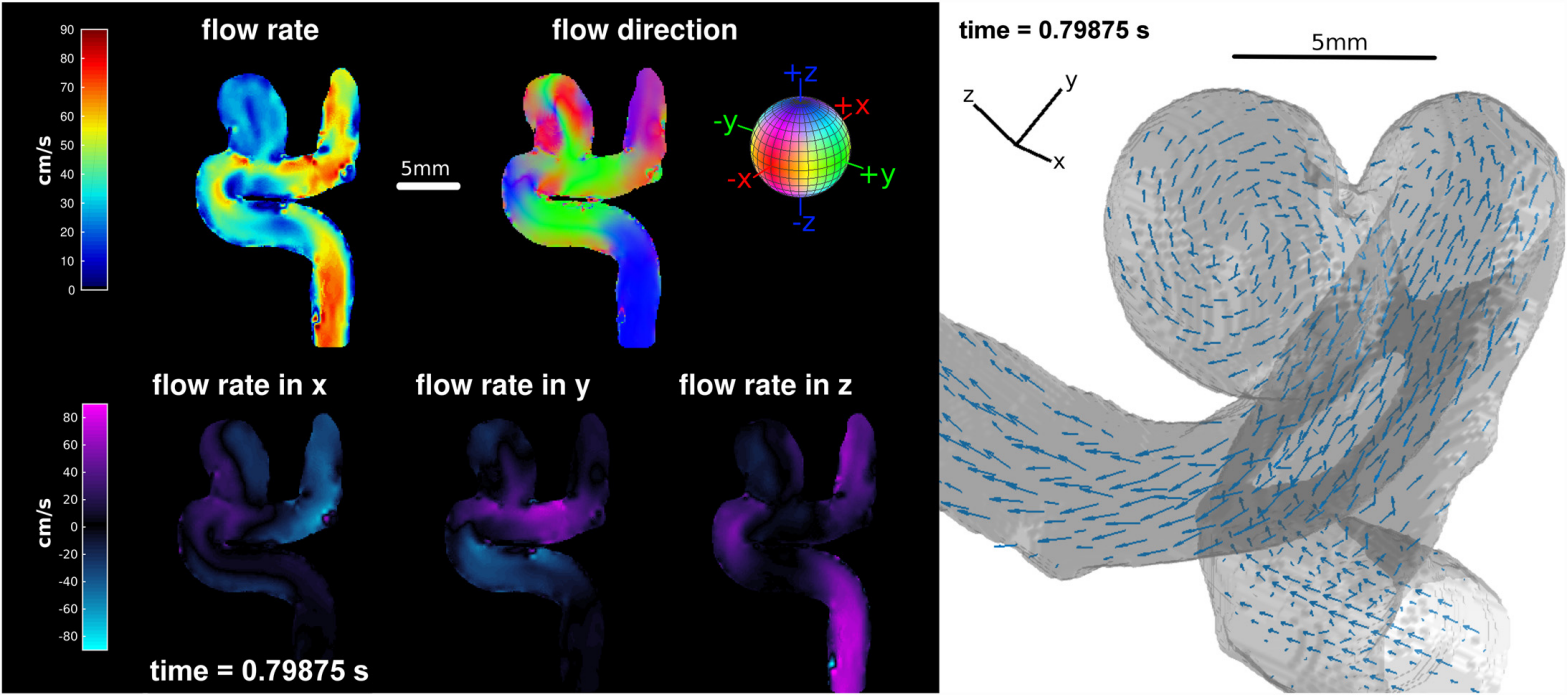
False color (left) and vector field (right) visualization of the 4D pc-fq MRI at the time point of maximal flow velocity during one pulsation cycle. Lower flow velocity and different flow directions are clearly visible inside the aneurysm. Movies showing all phases of the pulsation are available as S1 and S2 Videos.
